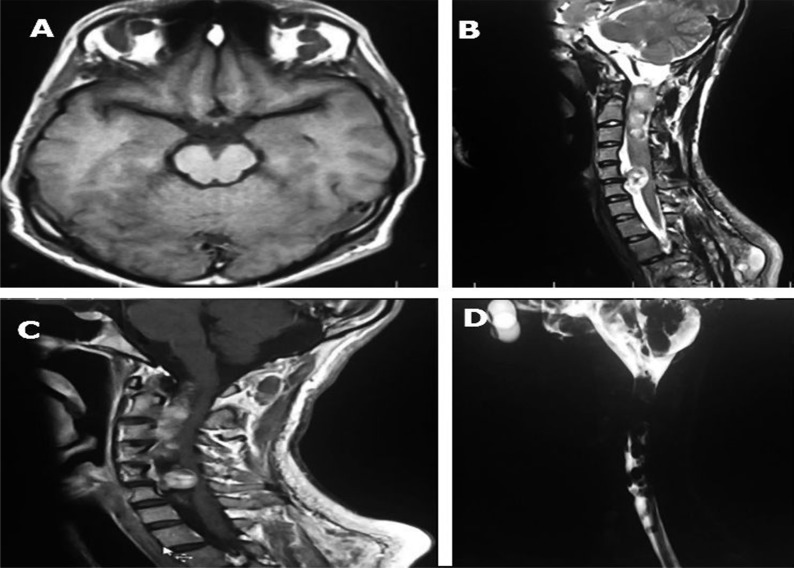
Widespread neurofibromas of orbital and cervical spine. Axial noncontrast T1-weighted image of brain reveals bilateral supra-orbital neurofibromas (A), Sagittal T2-weighted magnetic resonance imaging (MRI) of cervical spine reveals multiple intradural extramedullary neurofibromas, causing cord compression, widening of upper thoracic neural foramen and subcutaneous neurofibromas (B), Sagittal post-contrast T1-weighted image of cervical spine demonstrates multiple enhancing intradural extramedullary lesions causing cord compression (C), Sagittal cervical magnetic resonance myelogram ‎shows multiple intradural filling defects (D).
